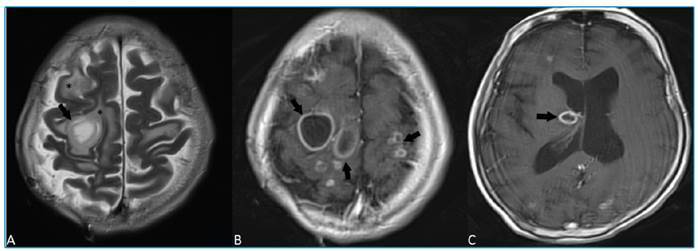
(A) Axial T2-weighted images showing hyperintense lesion (arrow) with surrounding edema (asterisk) of right frontal lobe. (B-C) Axial post contrast T1-weighted images showing ring-enhancing lesions (arrows) of bilateral parietal and frontal lobes, and right thalamus.
